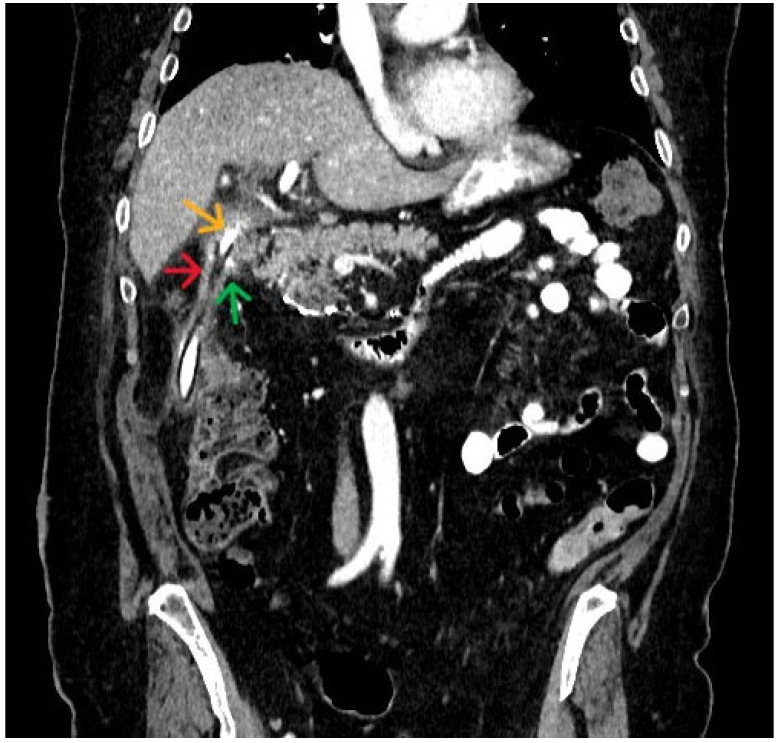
Abdominal CT scan with intravenous and oral contrast uptake demonstrating duodenum recanalization around surgical drain. Red arrow: surgical drain, Yellow arrow: recanalization of central duodenum, Green arrow: recanalization of peripheral duodenum.
